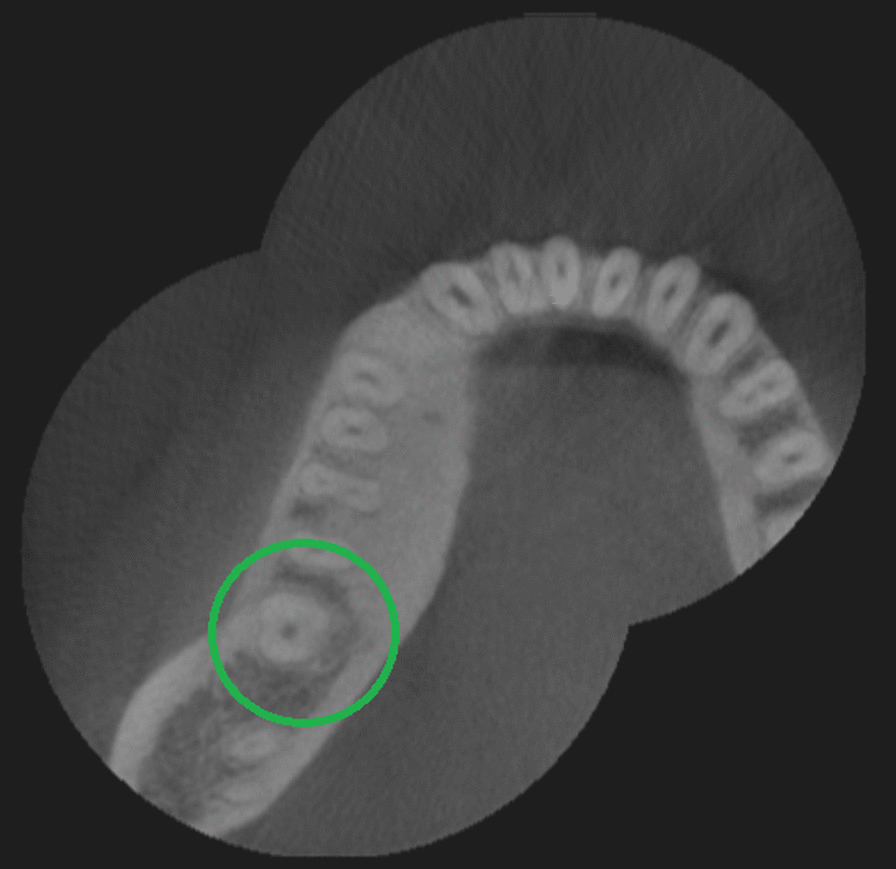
Axial view from CBCT scan showing mandibular second molar with one root
